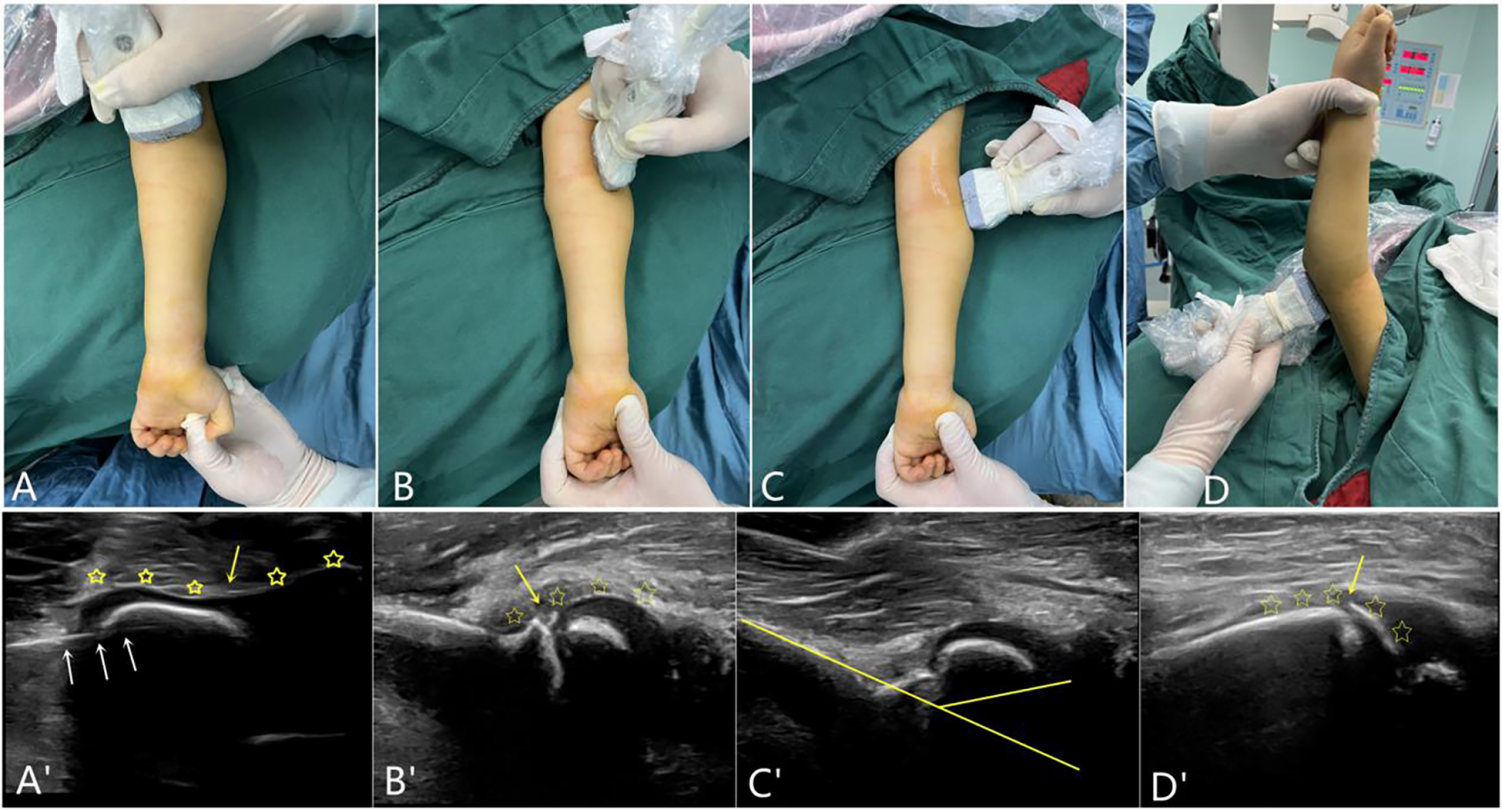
The probe position and corresponding scans on ultrasonography. (A) is the scanning probe position of the front coronal plane parallel to the articular surface. (A)’ shows no anteroposterior or lateral steps (yellow stars) or fracture line (yellow arrow) in the cartilage, and the white arrows indicate the k-wires. (B) indicates the scanning of the vertical articular surface of the anterolateral coronal plane. (B)’ shows no displacement of the fracture; the distal end of the condylar and the capitellum are continuous. (C) indicates the lateral sagittal plane vertical articular surface scan, (C)’ shows no displacement of the fracture; the condylar shaft angle was normal. (D) indicates the vertical articular surface scan of the posterolateral sagittal plane; the fracture line shown in (D)’ is essentially continuous.
